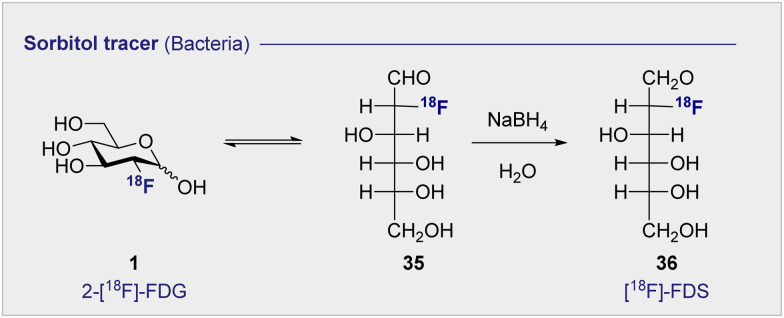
The synthesis of [^18^F]-FDS (36) by reduction of 2-[^18^F]-FDG (1).
